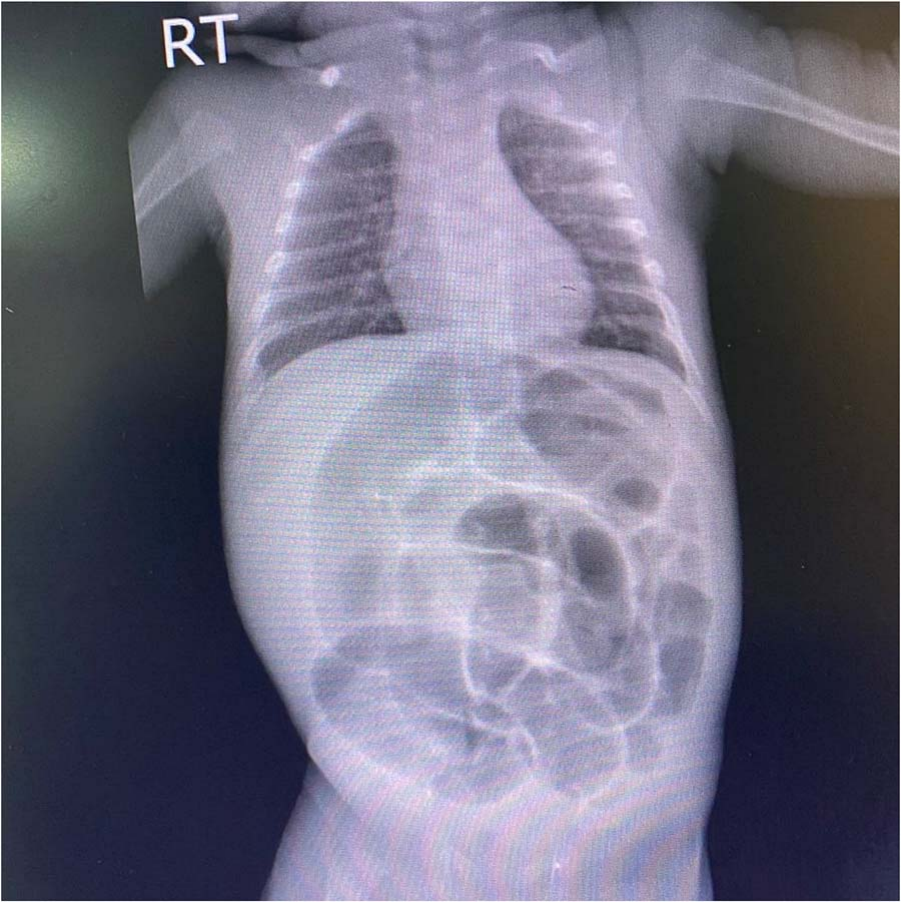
An anteroposterior abdominal X-ray of a male neonate shows marked gaseous distension of the abdomen with severe, diffuse dilation of numerous bowel loops. No evidence of pneumoperitoneum.
